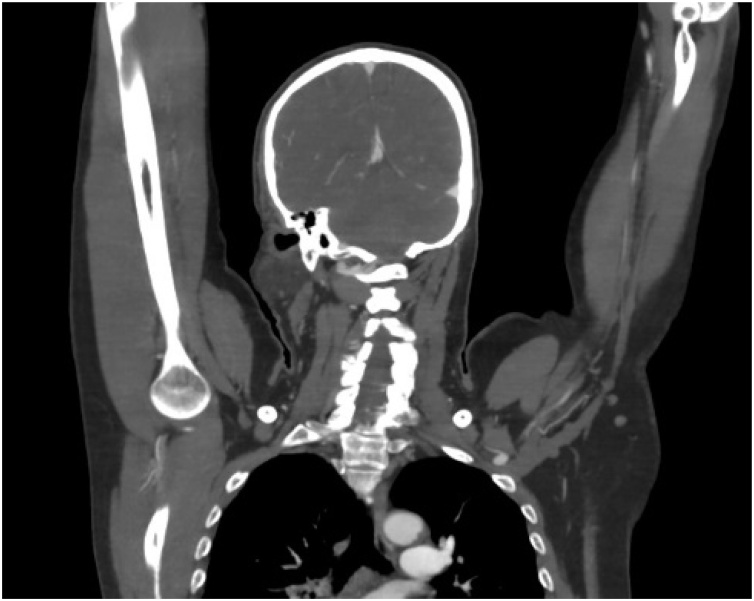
Left axillary node enlargement (white arrow) detected by chest CT scan including axilla with contrast at arterial phase.
